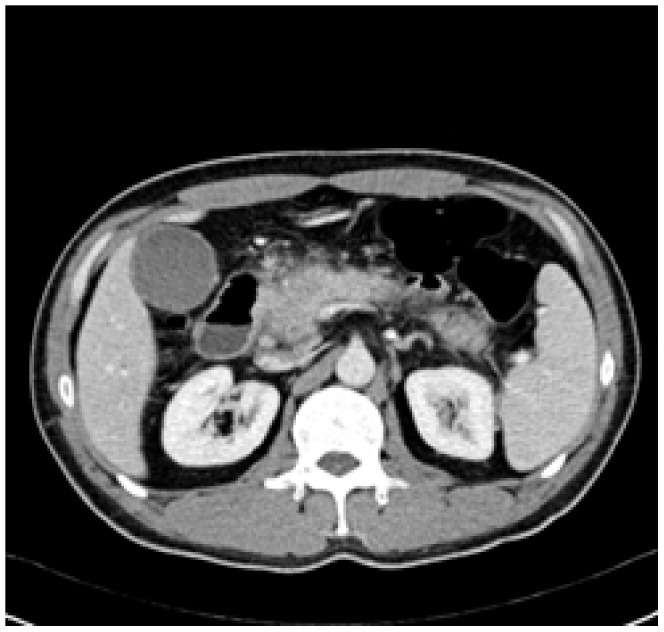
Enhanced CT showing nodules surrounding head of pancreas and interrupted common bile duct at the lesion.
